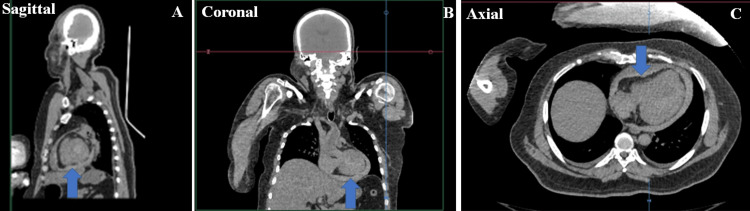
PMCT Images A, B, C: multiplanar reconstruction in PMCT showing the presence of hyperdense (white color) area separating the pericardium from the heart border suggestive of the presence of blood clots (blue arrow) PMCT: post-mortem computed tomography
